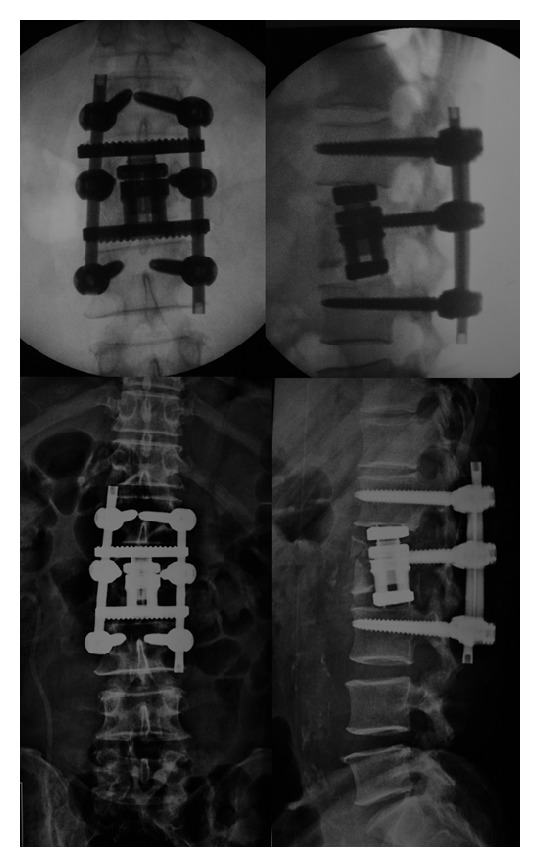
Intraoperative fluoroscopy and immediate postoperative X-ray showing implant position and fracture reduction.
